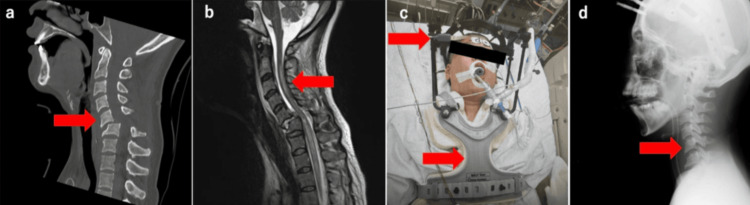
Imaging studies on the day of transport (a) CT scan showing anterior dislocation of C5 (red arrow). (b) T2-weighted MRI showing a high-intensity signal at the C3 level, indicating SCI (red arrow). (c) After endotracheal intubation and application of the halo vest (red arrow). (d) X-ray after manual reduction and halo vest application, showing reduction of the C5 dislocation (red arrow). SCI, spinal cord injury
